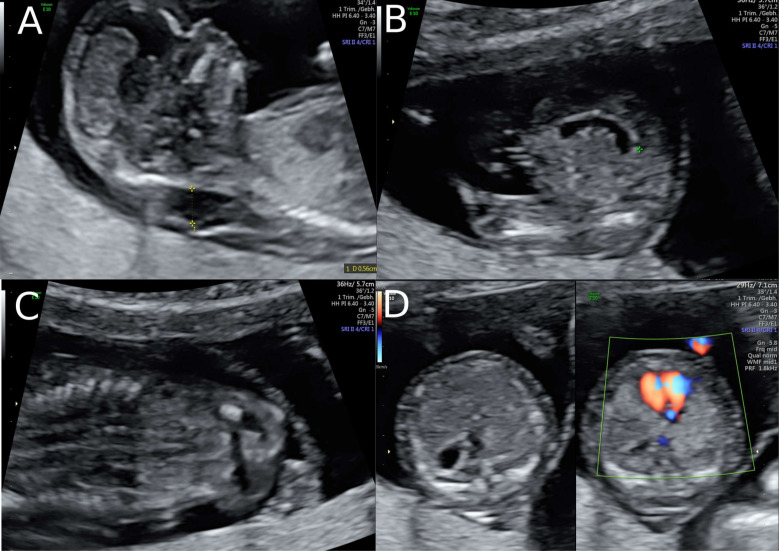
Ultrasound findings in the 1st Trimester. (A) Increased Nuchal Translucency (NT) at 13 + 5 weeks of gestation in twin II. (B) A simple cystic structure in the abdomen, most probably a gastrointestinal duplication cyst. (C) Hyperechogenic kidneys. (D) Malposition of the fetal heart in the chest at 13 + 5 weeks. The position of the fetal heart in the middle of the thorax.
